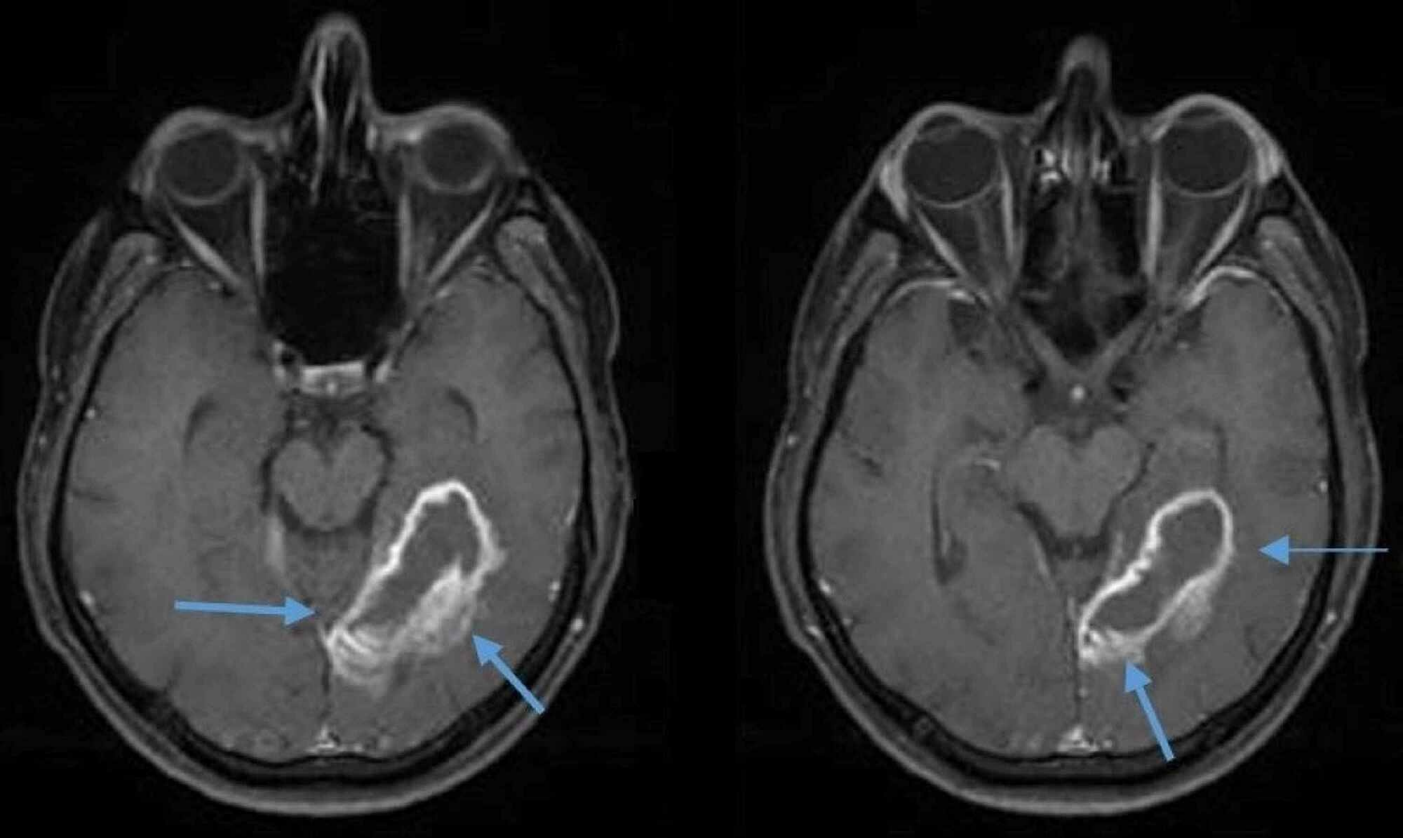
Axial images of the brain MRI showing a ring-enhancing lesion in the left occipitotemporal lobe with significant surrounding vasogenic edema (blue arrows). MRI: Magnetic resonance imaging
